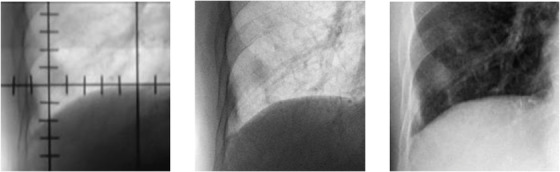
Fluoroscopy image (left), inverted dynamic chest radiography (DCR) image (center), and normal DCR image (right) of Patient #1
